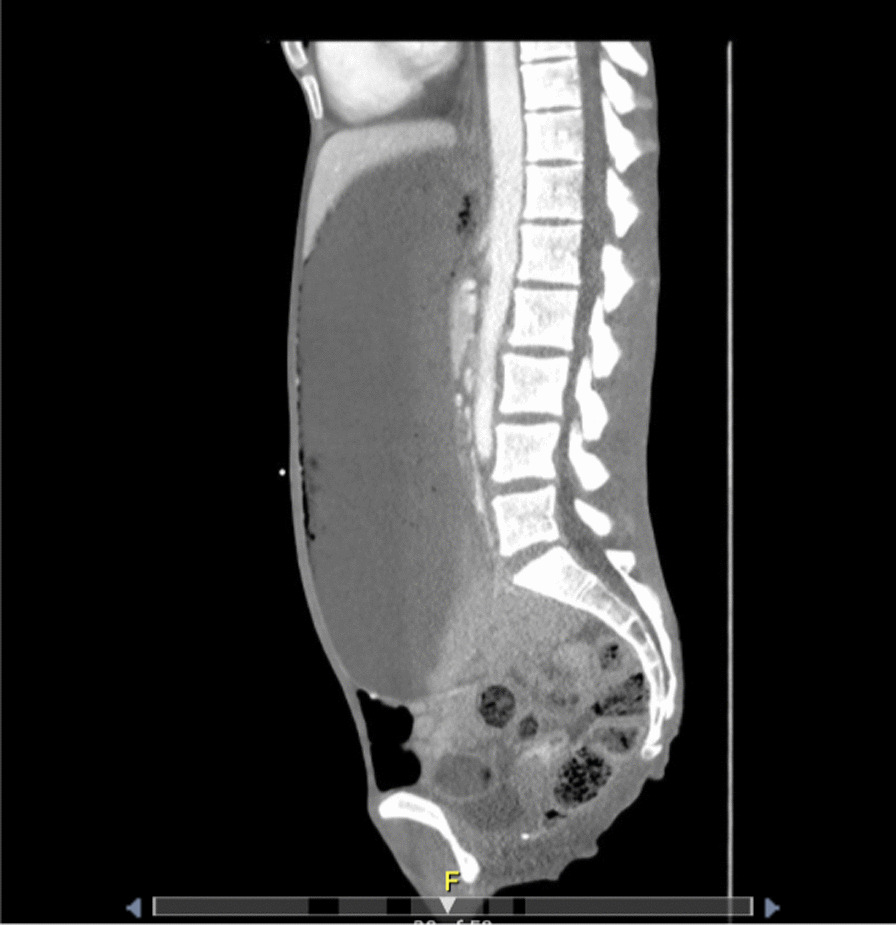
Computed tomography sagittal view
